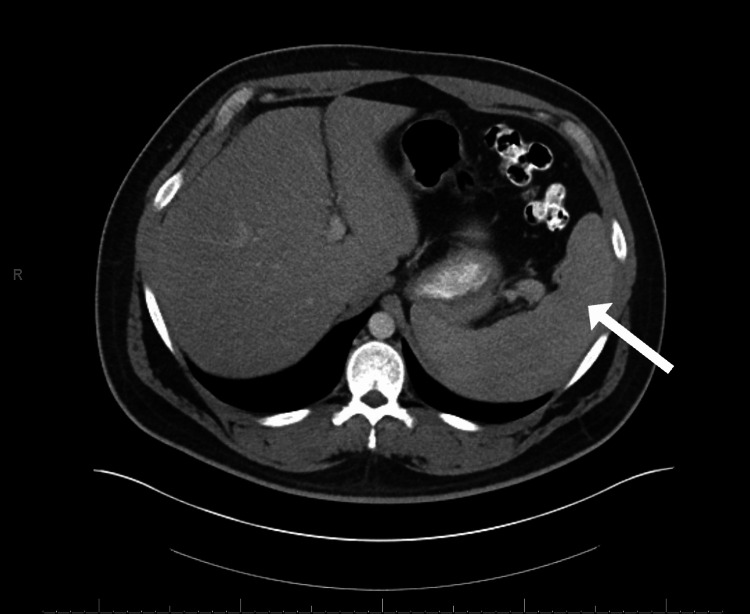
Transverse plane CT scan of abdomen showing improvement in infarcted areas (white arrow)
